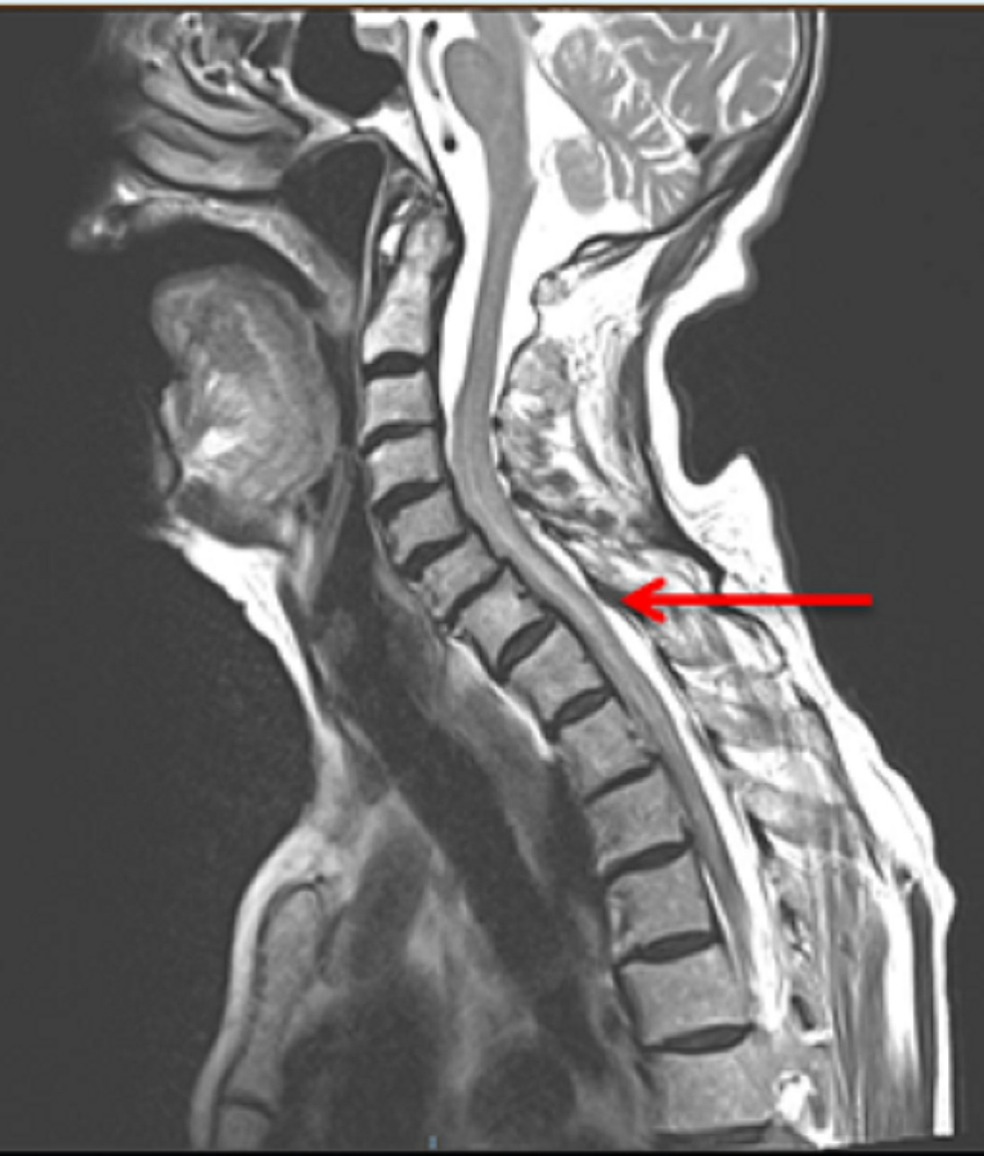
Sagittal T2-weighted sequence cervical and upper thoracic spine. The spinal subarachnoid haemorrhage is demonstrated as abnormal low signal intensity posterior to the T1 vertebral body (red arrow). It is important to view the T1 and T2 images together. Blood can be of varying signal intensity depending on the acuity of the haemorrhage as different stages of blood products have varying paramagnetic qualities.
